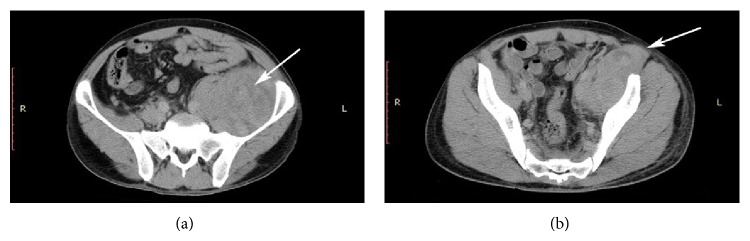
Abdominal CT showed a large retroperitoneal hematoma, which extends through femoral triangle. White arrows in the pictures highlight left iliopsoas hematoma and its inguinal extension.
